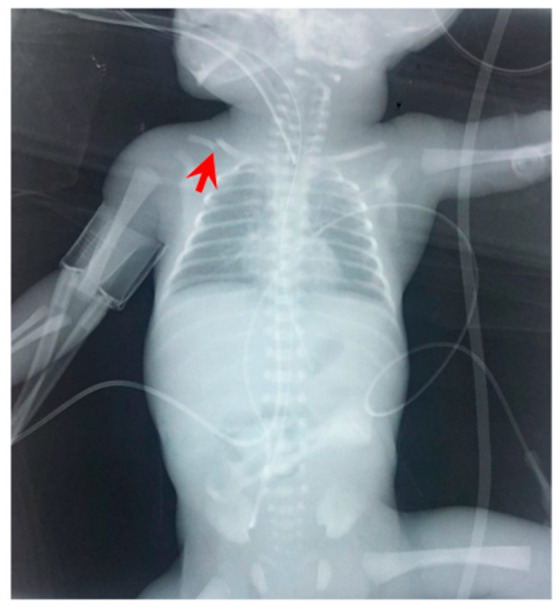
Radiography of the neonate on the day of deterioration. Non-specific signal characteristics in the abdomen, such as distention and poor distribution of bowel gas. A clavicular fracture clavicle is noted on the right (red arrowhead).
